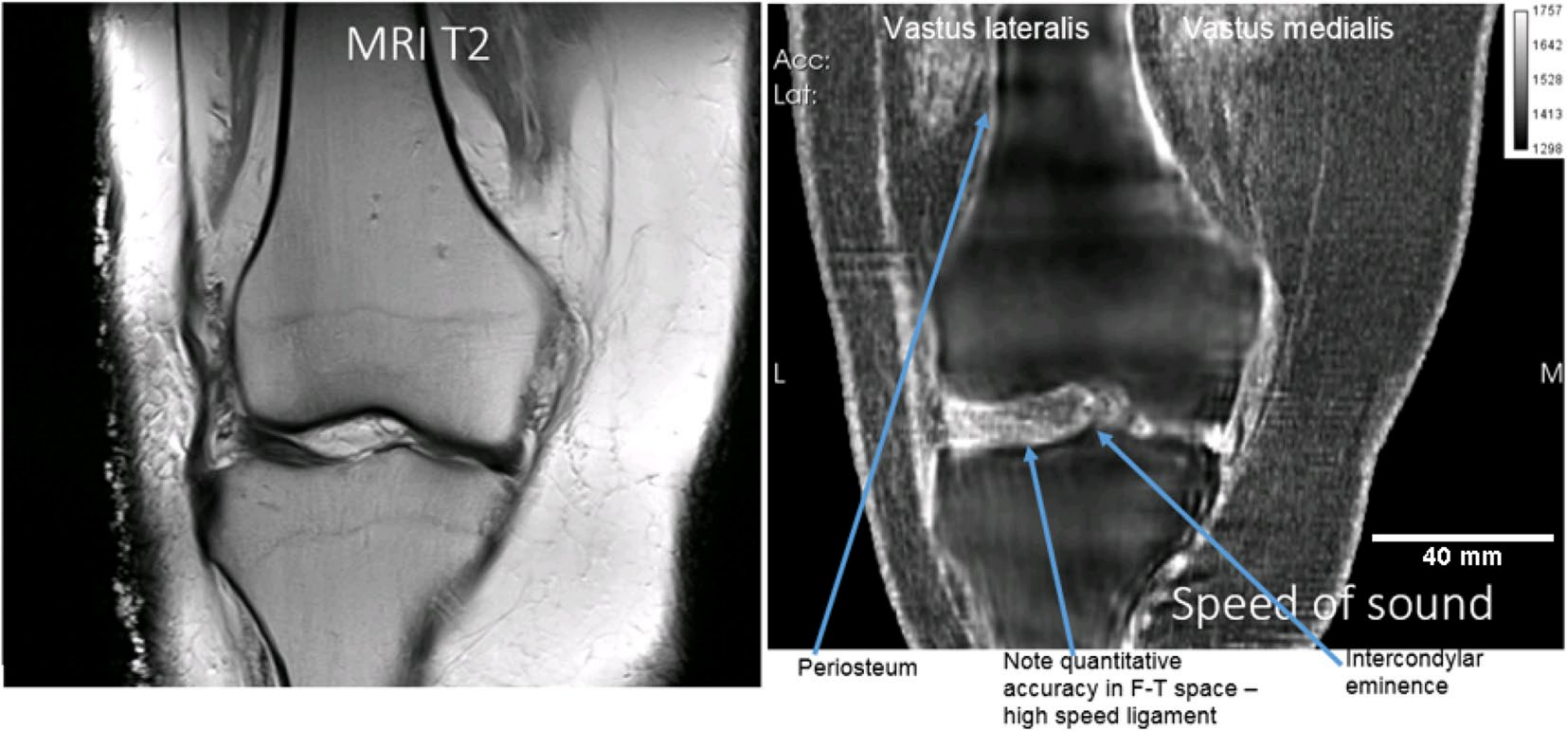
Showing correlation of speed of sound (right) and MR images (left) for the coronal view of the cadaver knee for T2 weighted MR image on the left versus SOS image on the right. Note the periosteum has the correct high speed and is clear on the QT ultrasound SOS image (right) whereas it does not generate a signal in the MR image (left panel). The lateral collateral ligament is likewise visible with correct SoS on the right but does not generate a signal in the MR image (left).
